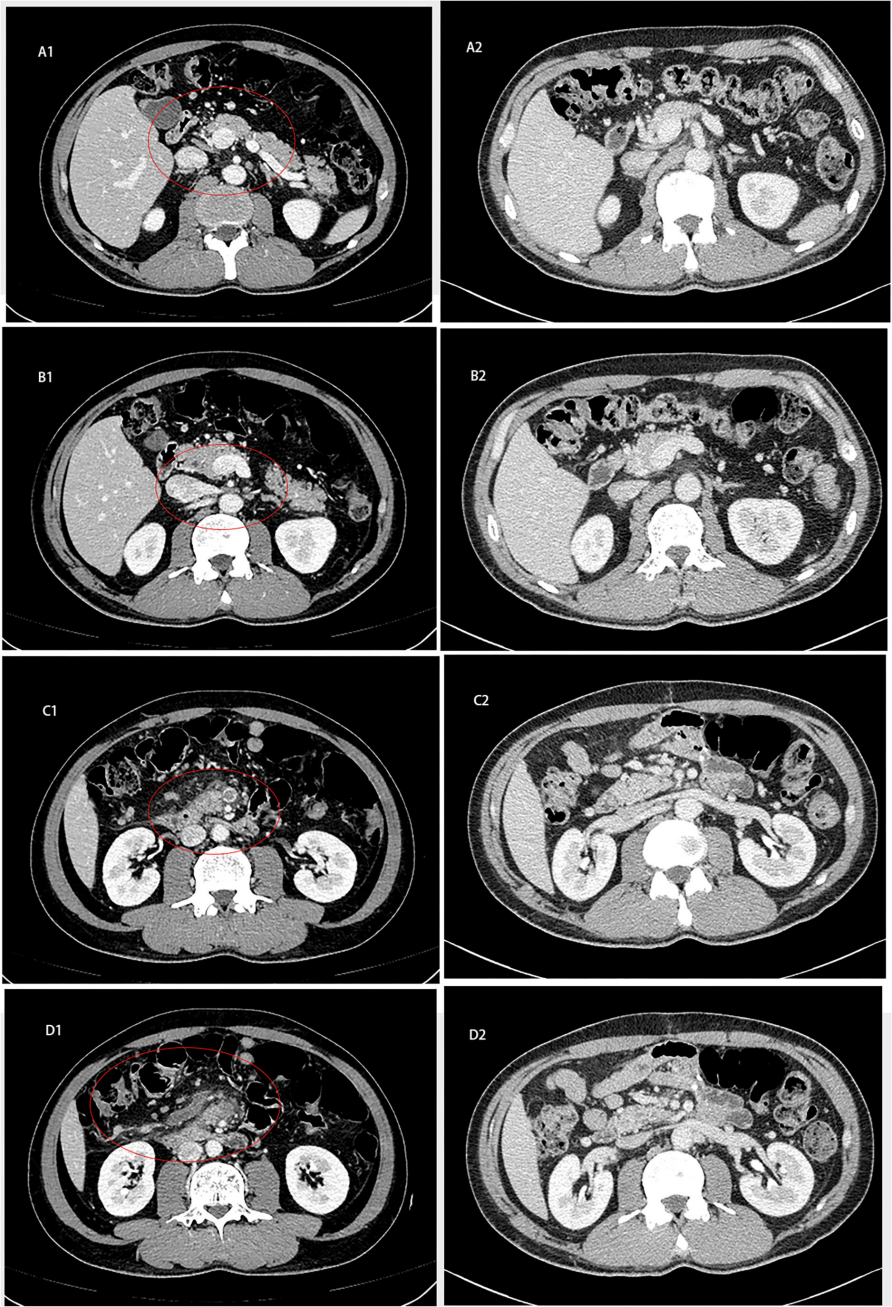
Abdominal computed tomography of patient. (A1–D1) Contrast-enhanced CT on February 2, 2020 showed that the small intestine in the right middle abdomen was occupied by tumor with multiple enlarged lymph nodes. (A2–D2) Abdominal CT on October 6, 2020 showed that the obstruction of intestinal lumen was relieved after operation 2020.
